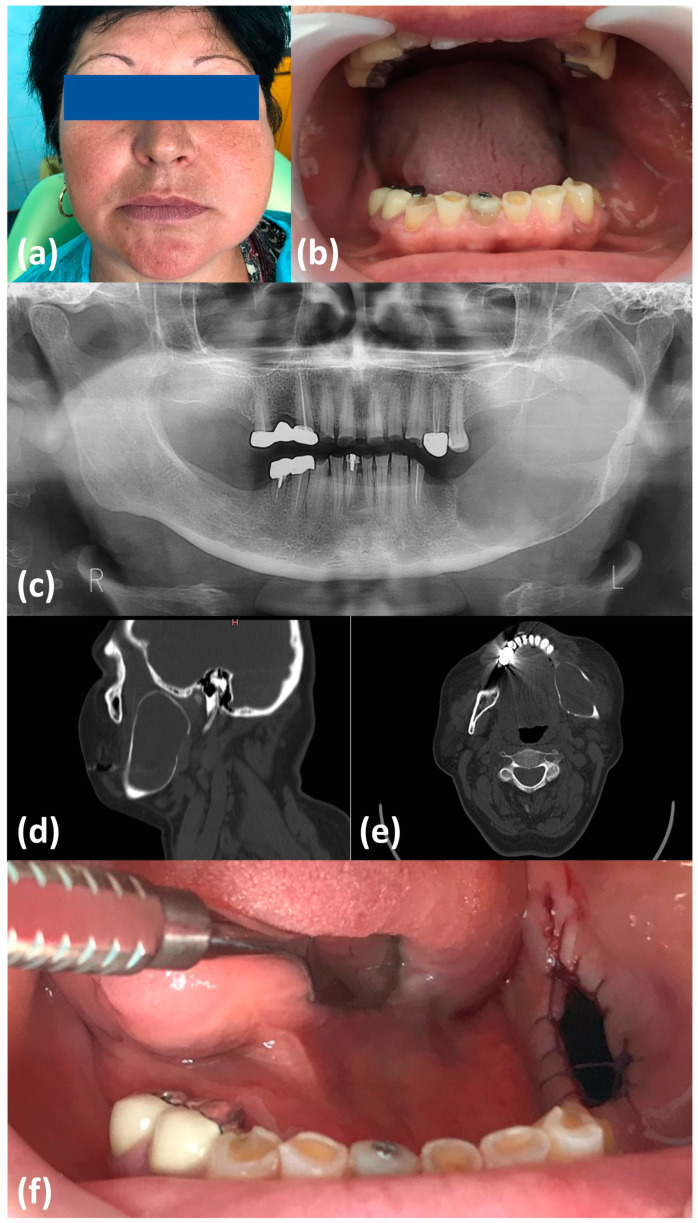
Clinical and imagistic aspect at presentation. (a) Extraoral aspect; (b) intraoral aspect; (c) preoperative panoramic X-ray; (d) preoperative native CT scan: sagittal view; (e) axial view; (f) intraoral aspect after marsupialization of the cyst.
